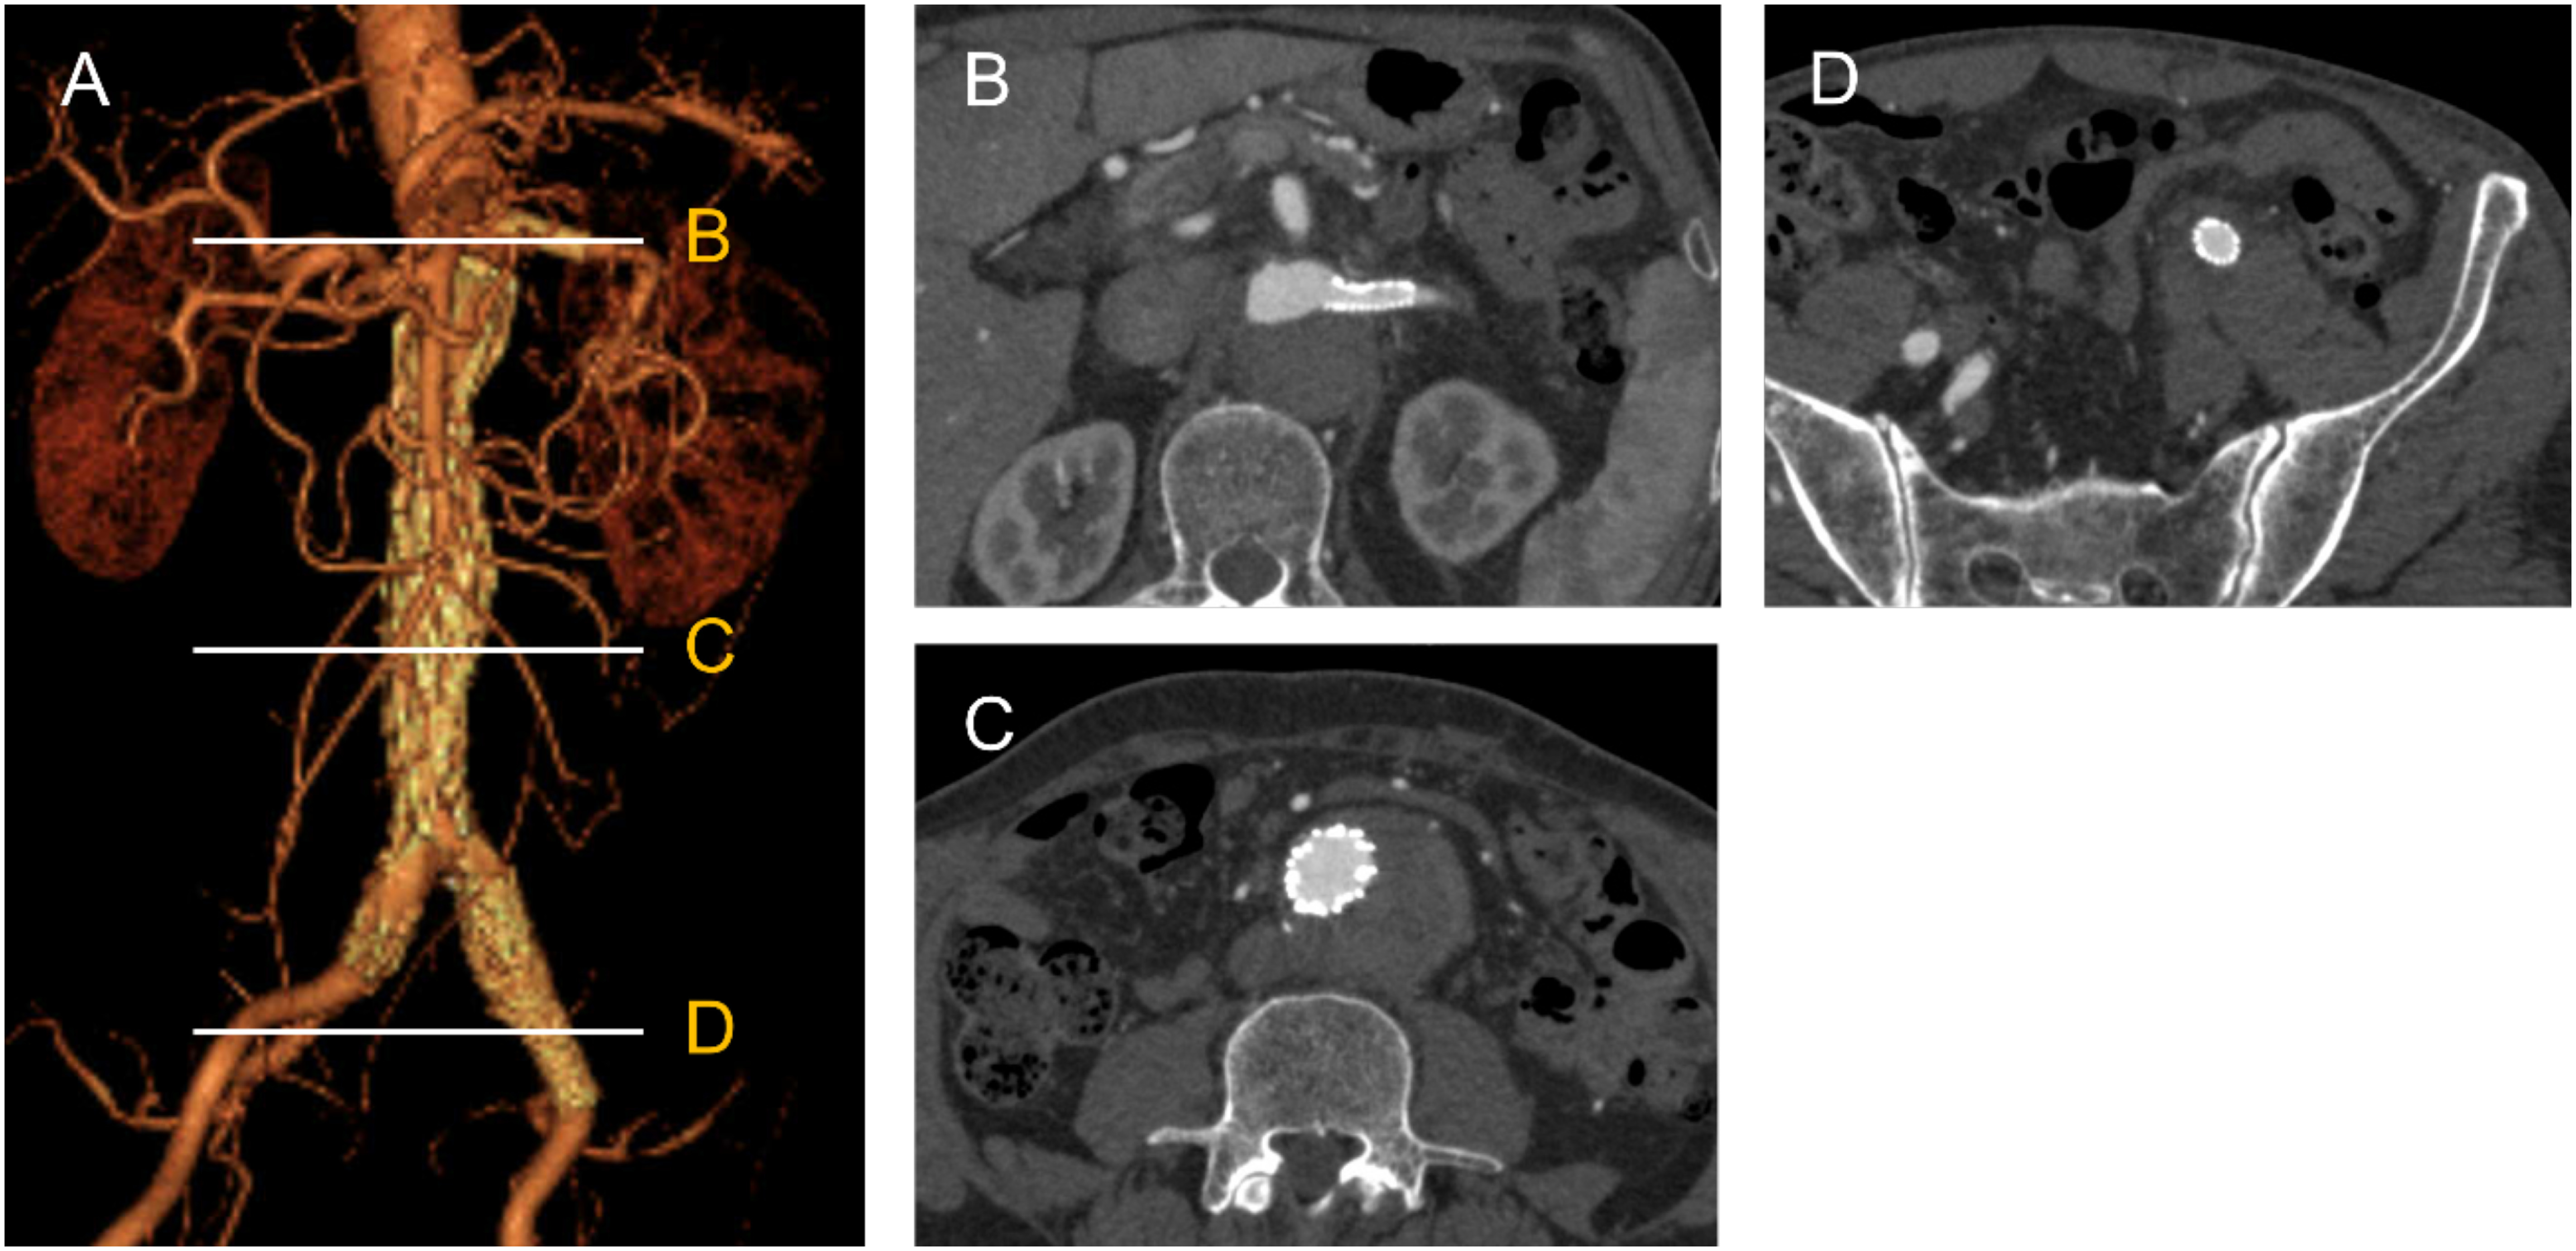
Fig. 3 Contrast-enhanced computed tomography 1 month after the procedure showing no false lumen flow without endoleaks (A–D).
